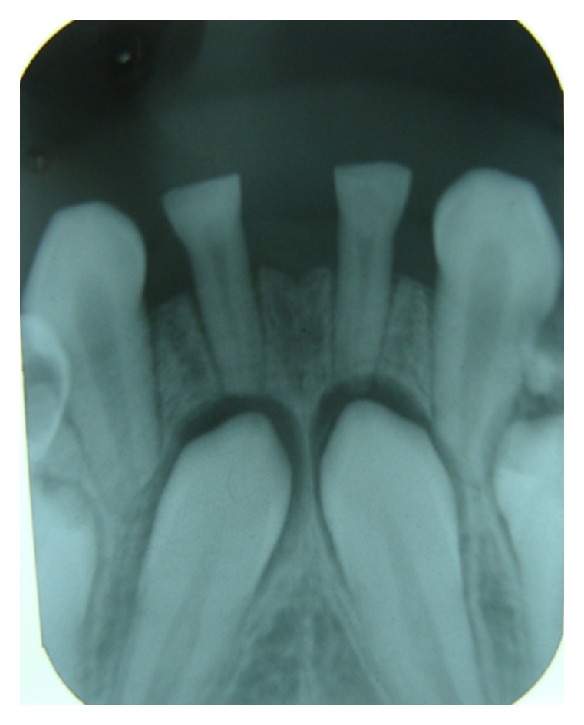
Periapical radiograph shows missing permanent mandibular incisors.
